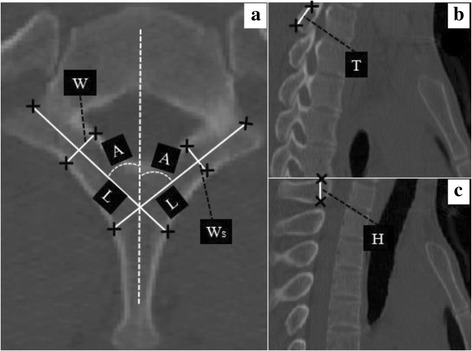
Measurements of T1 on a transverse computed tomography (CT). a L: length based on the ideal laminar screw trajectory. A: the axial angle between the ideal laminar screw trajectory and the midline. W: the minimal outer cortical width. Ws: the minimal cancellous width. b Minimal outer cortical width (T) measured in the sagittal reconstruction at the middle 1/3 narrowest lamina. c The spinous process height (H) obtained at the site of the cross-point of the bilateral ideal laminar screw trajectory in the coronal plane
